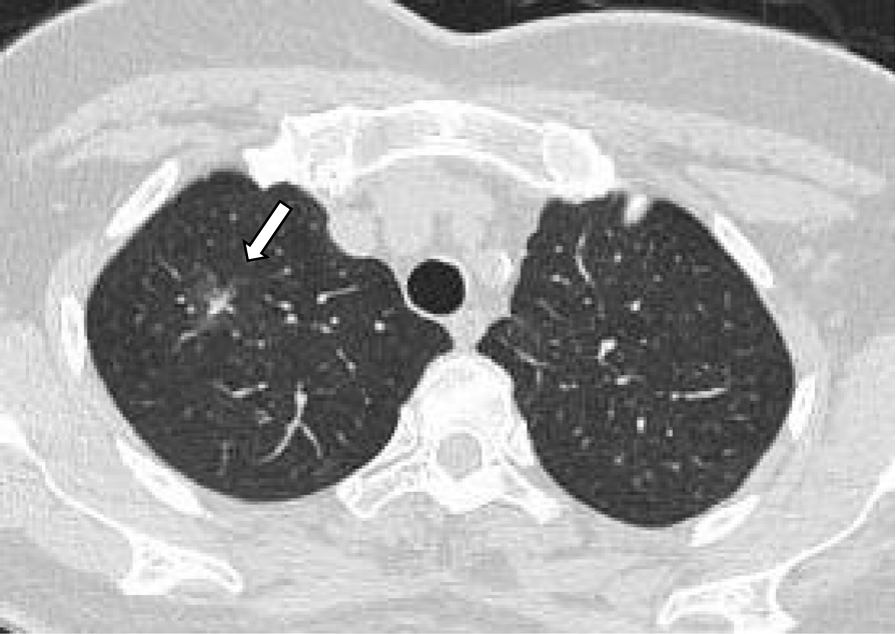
Ground-glass opacity (GGO) with ALK rearrangement. Axial CT image in a patient with ALK-positive adenocarcinoma showed a pure-GGO (arrow) in the right upper lobe with vessel underlying
